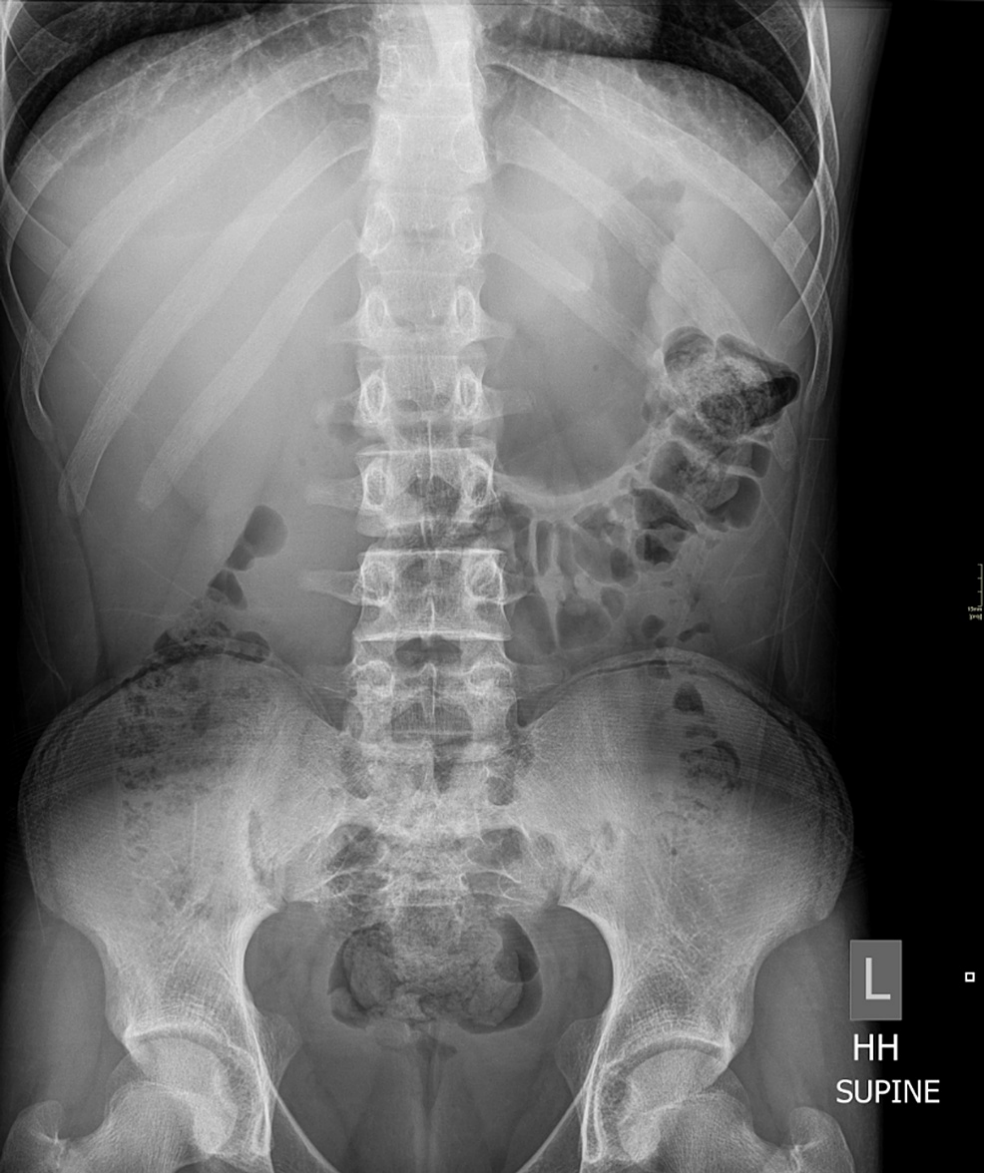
Conventional radiograph, supine abdomen, and pelvis (- 70 KV, 100 mAs) showing no calcifications
